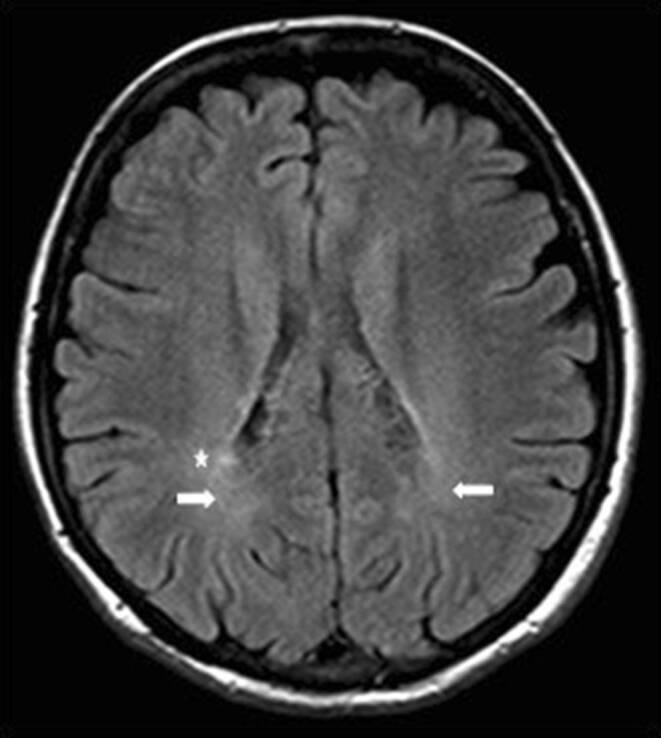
Dirty appearing white matter (DAWM) in a patient with PPMS. Fluid attenuated inversion recovery (FLAIR) image in the axial plane. Around the posterior horns of the lateral ventricles, ill-defined areas of increased signal intensity are visible (*white arrow*). Right periventricular hyperintense focal lesions are also visible (*asterisk*)
